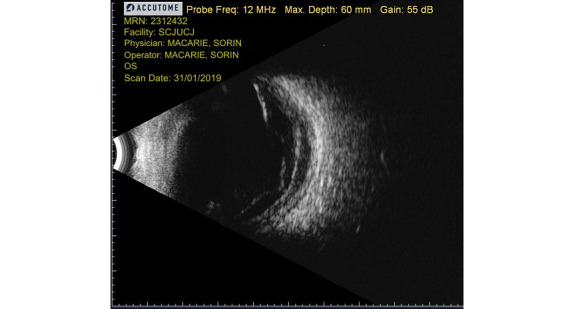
B-scan ultrasound, left eye
